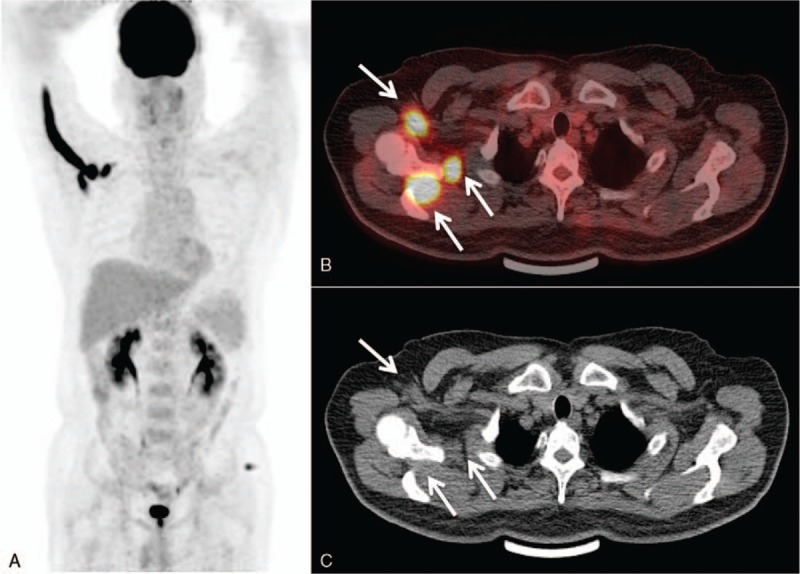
Follow-up PET/CT after radiotherapy. PET MIP (A), axial fusion image (B) and axial CT (C) revealed intense FDG uptake in the brachial plexus, axillary, suprascapular and median nerve with SUVmax of 19.9, suggesting recurrence of lymphoma.
